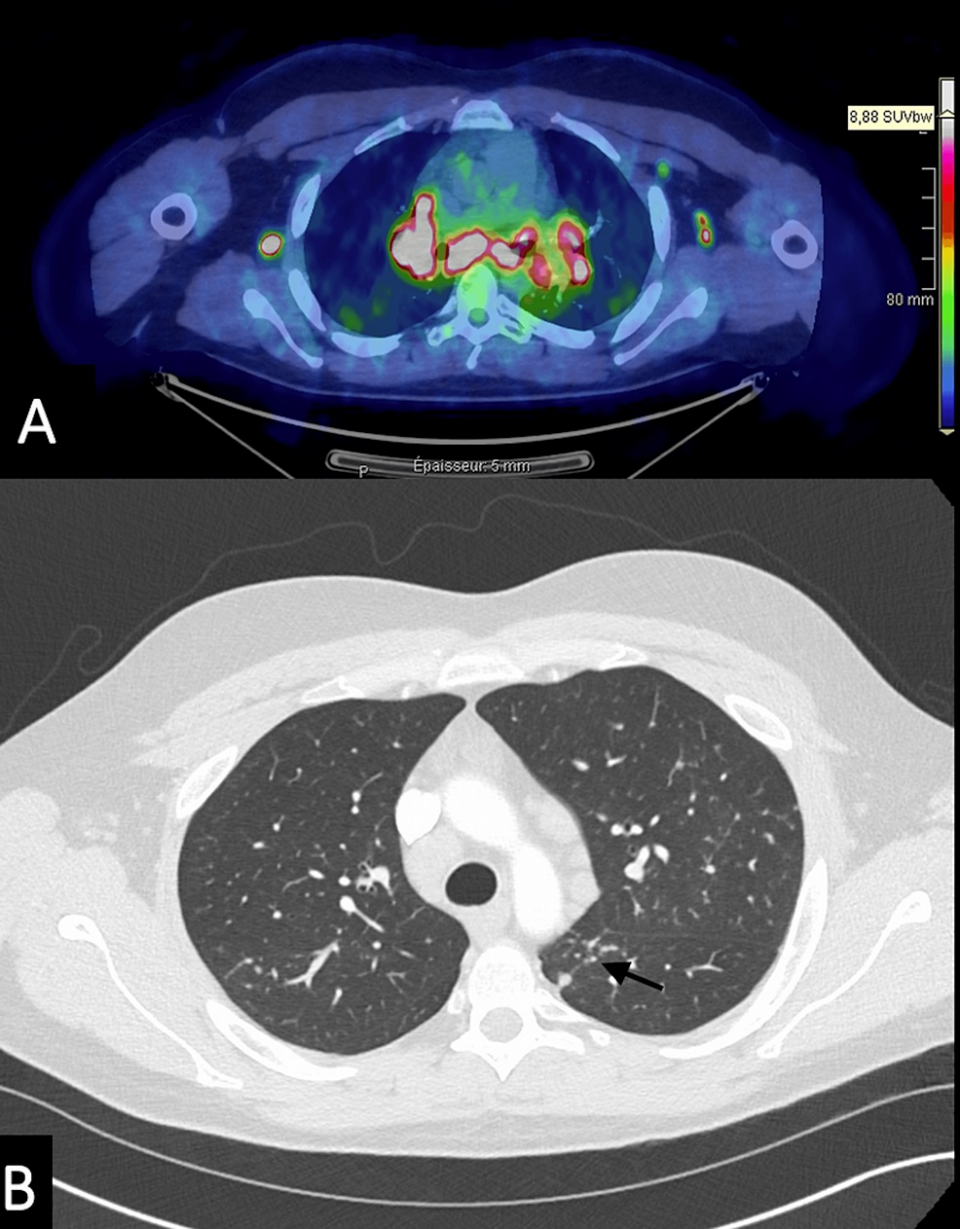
Multiple mediastinal, hilar, retroperitoneal, and inguinal hypermetabolic lymphadenopathies Axial FDG-PET/CT (A) shows in white mediastinal, hilar, and axillary lymphadenopathies with an elevated SUV. Axial chest CT (B) shows nodular and micronodular involvement of the subpleural and peribronchovascular regions in the upper segment of the left lower lobe (black arrow). The SUVmax was 8.88. FDG-PET/CT: fluorodeoxyglucose-positron emission tomography/computed tomography, SUV: standardized uptake value, CT: computed tomography, SUVmax: maximum standardized uptake value
